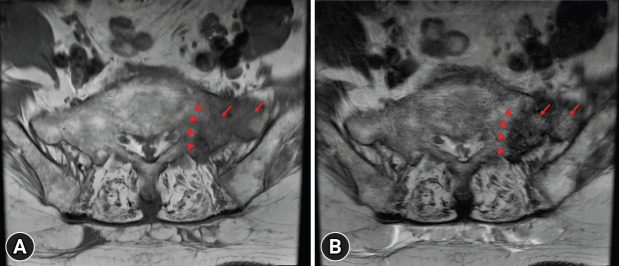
(A) Axial T1-weighted and (B) axial T2-weighted pelvic magnetic resonance imaging shows low T1 and high T2 signal intensity in the left sacral ala, indicative of bone marrow edema (arrows). Additionally, a hypointense fracture line (arrowheads) is visible laterally to the sacral foramina, consistent with sacral insufficiency fracture.
